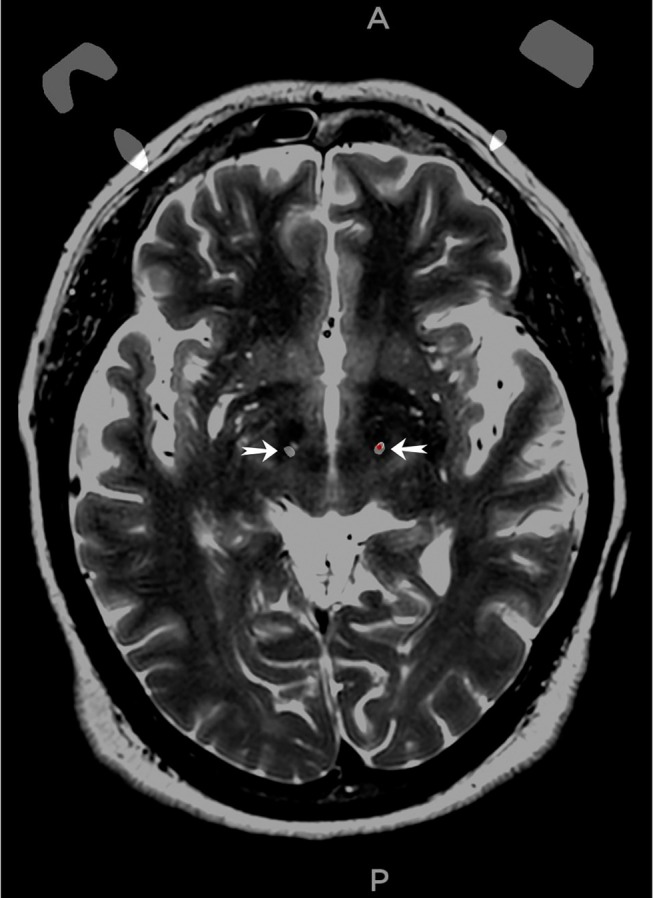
The merged T2 sequence of magnetic resonance imaging and post‐op computed tomography scan of the patient indicates the location of leads. The pointer in each site indicates the location of the lead.
